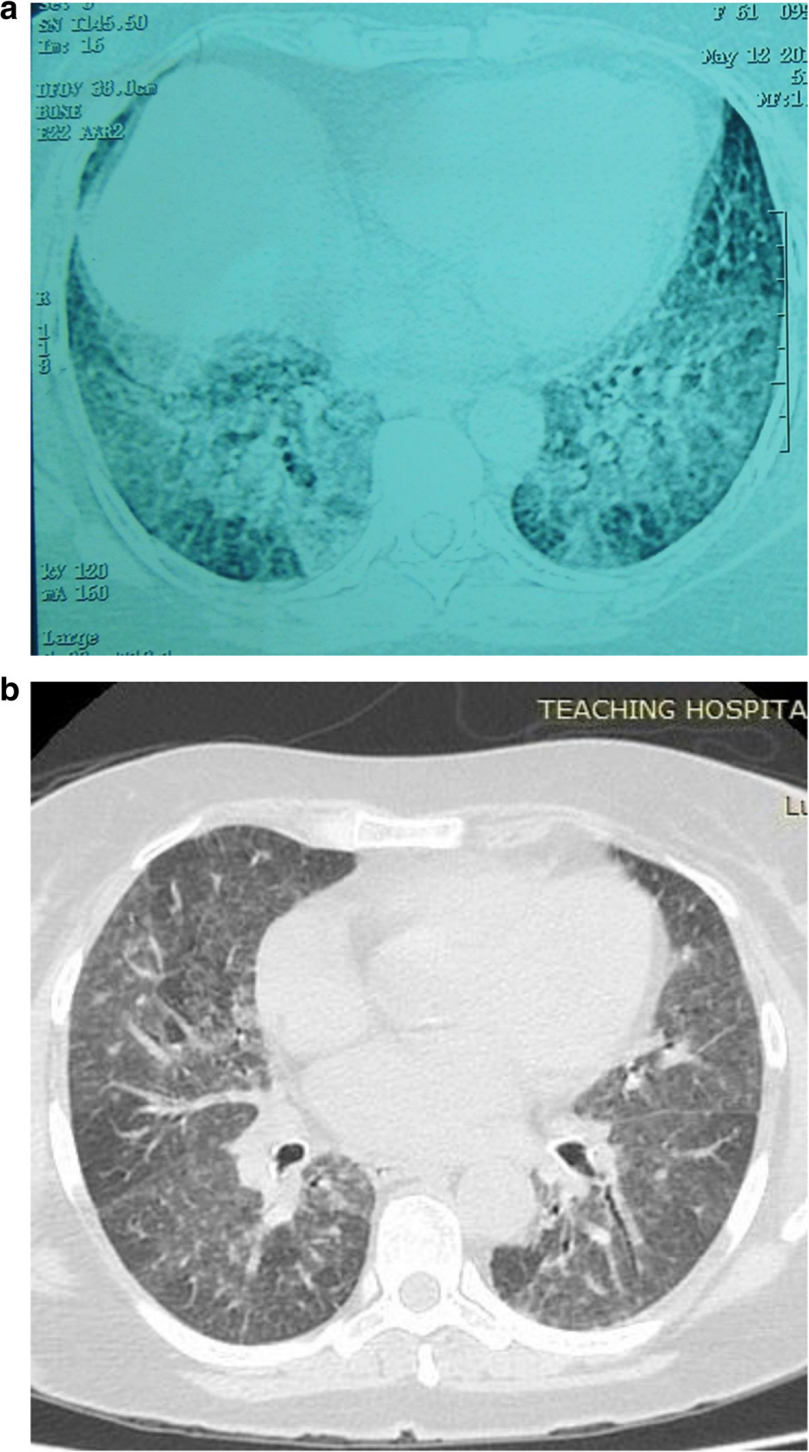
High-resolution computed tomography scans. (a) A high-resolution computed tomography scan of the chest (before the lavage) showing septal thickening with ground-glass shadows more on bilateral mid and lower zones. (b) A high-resolution computed tomography scan of the chest (after the lavage) showing significant resolution of the bilateral septal thickening with minimal resolution of ground-glass opacities.
